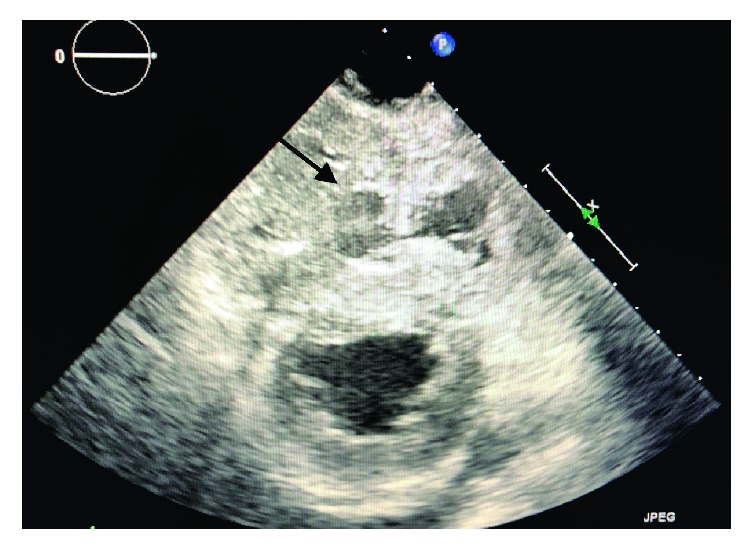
Transesophageal echocardiogram with TG SAX view showing extensive thrombus formation in the right ventricle.
